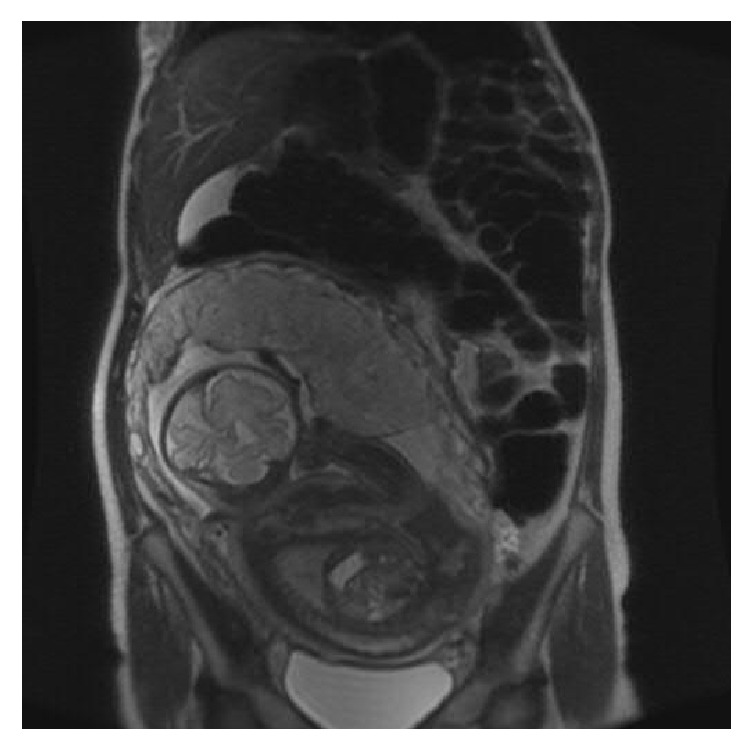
Magnetic resonance imaging without contrast performed at 32 weeks' gestation. There is marked distention of the sigmoid colon beginning at the level of the sigmoid along the left lateral aspect of the uterus where there appears to be a small, early (90 degree) twist of the sigmoid colon. The fetus may also be seen in this image.
